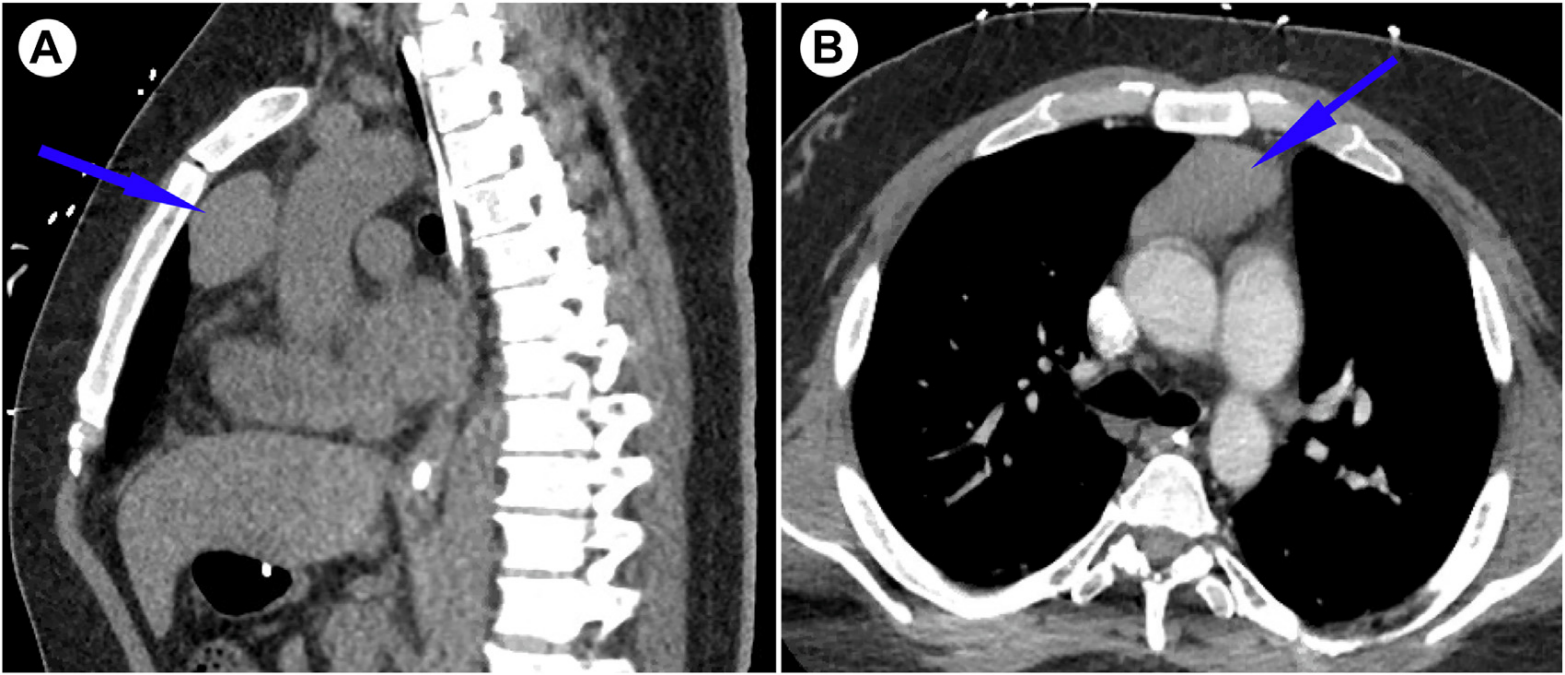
(A) Computed tomography sagittal section of the thorax shows thymoma (blue arrow) in the anterior mediastinum. (B) Transverse section of the same.
